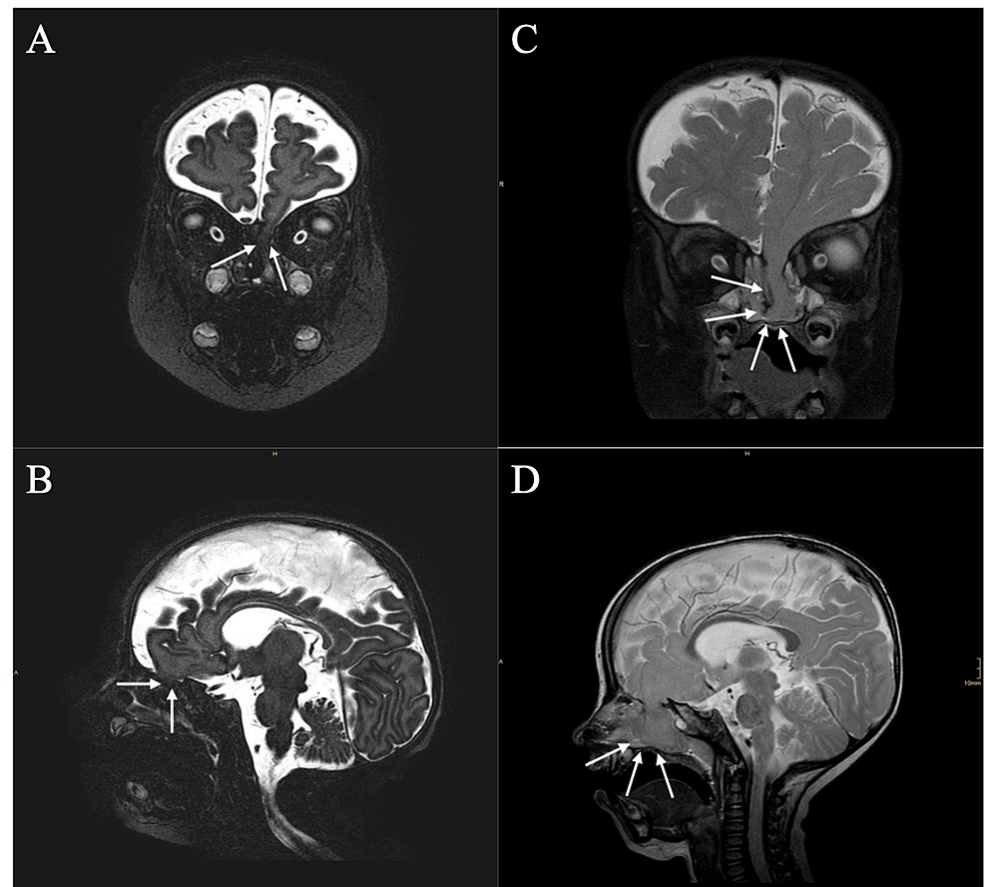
Strict MRI controls with coronal and sagittal T2-weighted sequences (A) and (B) approximately two months after birth, showing the bone defect of the anterior skull base with the concomitant meningoencephalocele (white arrows), (C) and (D) approximately six months after birth, displaying the assumed increase of the diameter of the bone defect as well as an enlarged meningoencephalocele (white arrows). MRI: magnetic resonance imaging.
